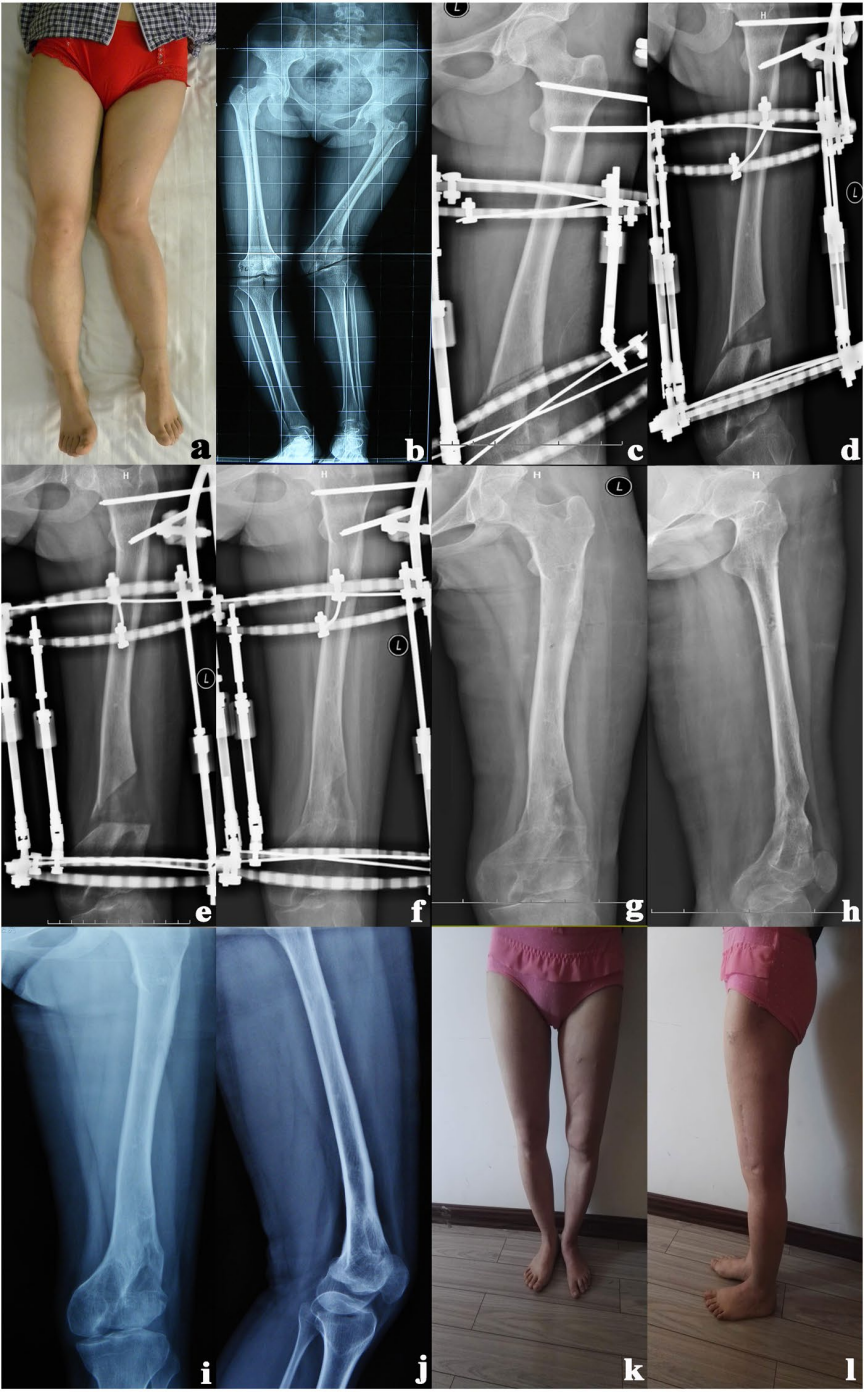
A 20-year-old female patient had short femur with severe genu valgus. (a) Short femur with severe genu valgus of the left lower limbs before operation. (b) Preoperative X-ray scan: the left femur was 30 mm short, FTA:138°, aLDFA:43°. (c) The osteotomy plane should be parallel to the distal femoral articular surface. (d) extended for 14 days and the lateral extension pole was adjusted to 28 mm. (e) Extended for 32 days and the lateral extension pole was adjusted to 64 mm. (f) The callus of the extended part was mature after lengthening for 8.5 months. (g,h) The external fixator was removed. (i,j) The osteotomy showed no loss. k, l: the anterior and lateral force lines of the left lower limb were good postoperatively after 4 years.
